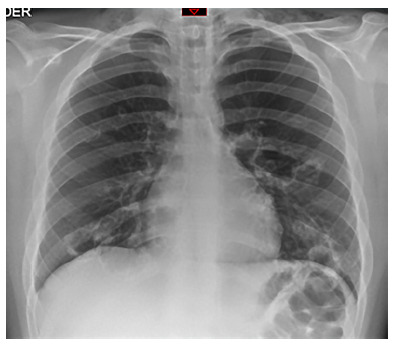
Chest X-ray. The image shows cervical subcutaneous emphysema, multiple cystic formations in both lungs, measuring approximately 38 x 26 mm as well as discrete pneumomediastinum.
